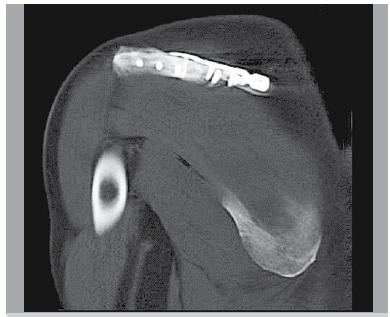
The 2D CT image of radiographic union at three months follow-up.
